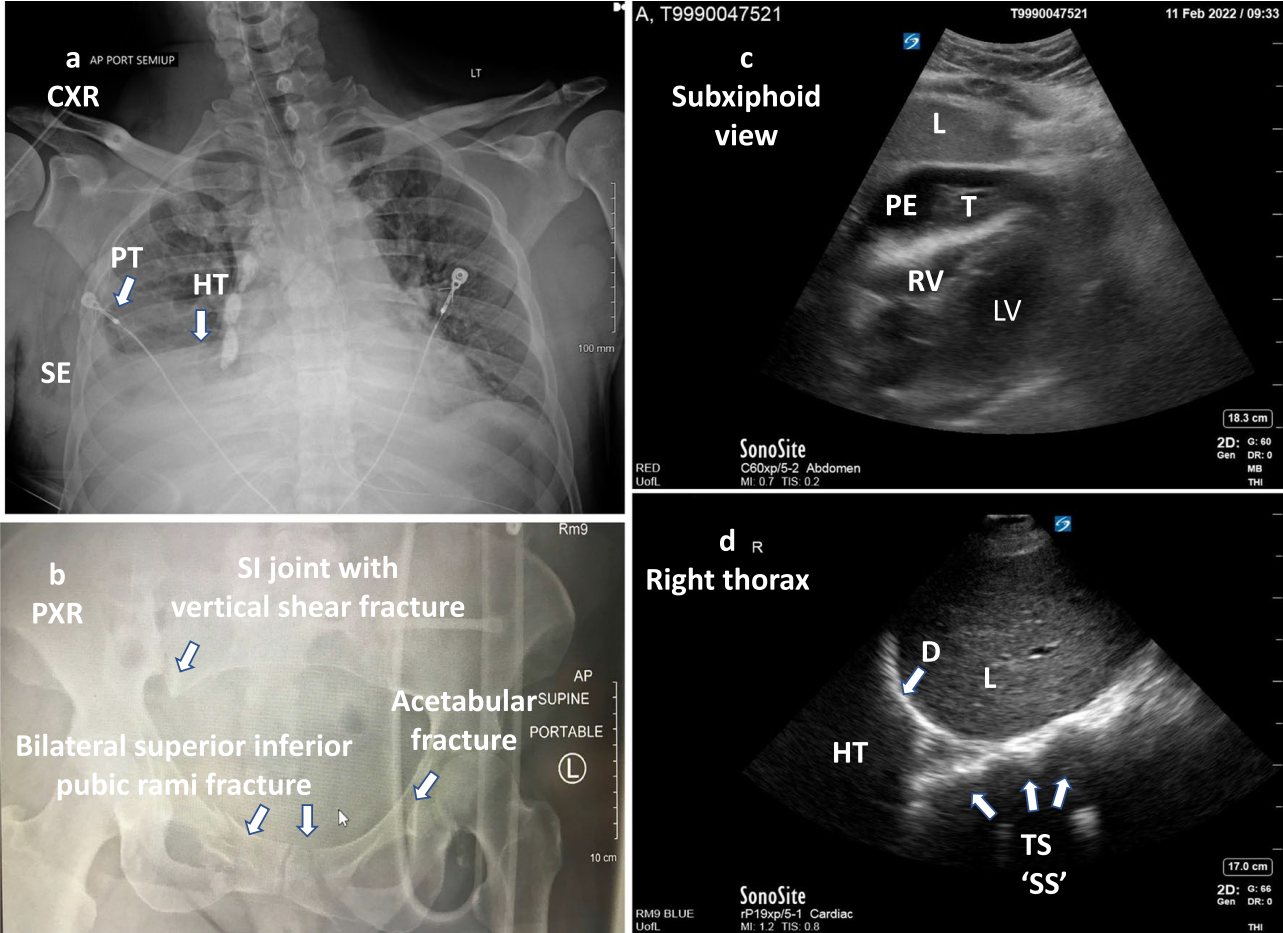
a Chest X-ray AP view. Right sided hemothorax, right lateral pneumothorax and subcutaneous emphysema. b Pelvic AP view. Widening of right sacroiliac joint with right sacral fracture and vertical shift (potential vascular injury), bilateral superior inferior pubic rami fracture (risk for bladder injury), left acetabular fracture. c Subxiphoid view of the heart (2D). Large pericardial effusion causing tamponade. d Right thoracic view at the diaphragm with a right hemothorax. Thoracic spine visualized above the diaphragm (spine sign). Normally, the thoracic spine is obscured by air within the lung. *D* diaphragm, *HT* hemothorax, *L* liver, *LV* left ventricle, *PE* pericardial effusion, *PT* pneumothorax, *RV* right ventricle, *SE* subcutaneous emphysema, *SI* sacroiliac joint, *SS* spine sign, *T* thrombus, *TS* thoracic spine
